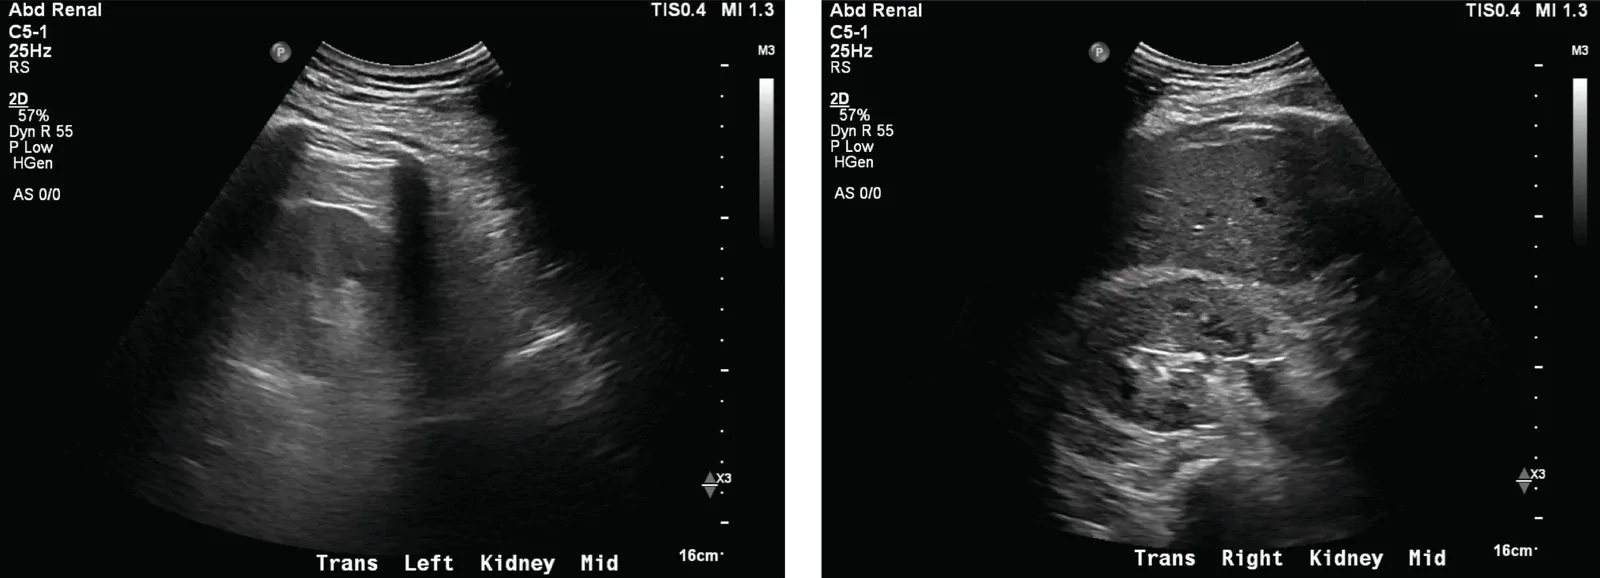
Renal ultrasound images obtained on admission Transverse views of the left and right mid kidneys through the renal pelvis. Ultrasound revealed mild bilateral hyperechogenicity with no hydronephrosis.
